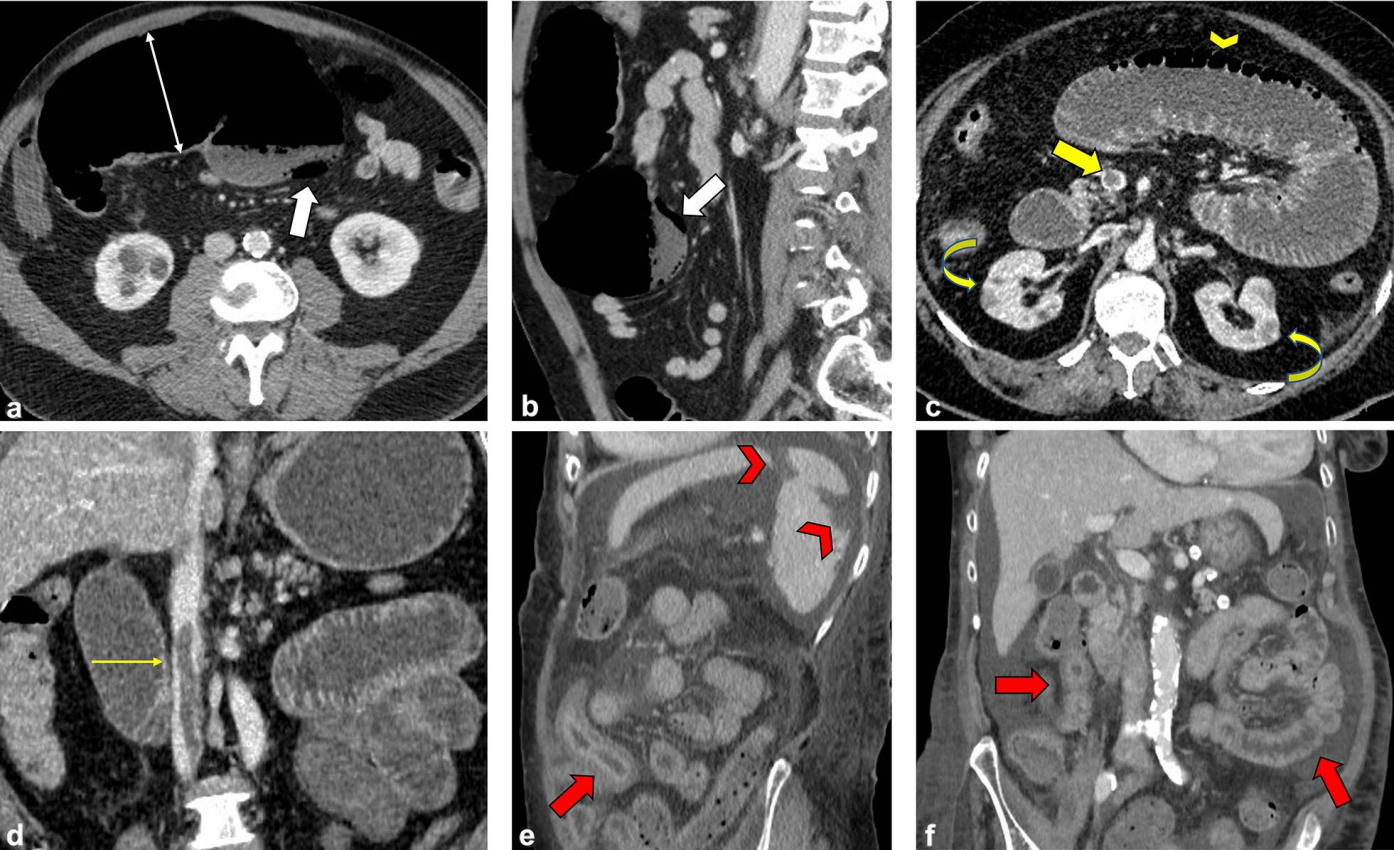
Representative images of abdominal ischemic complications. Computed tomography scans from COVID-19 patients with ischemic complications. A, B Axial and sagittal CT images of a 63-year-old patient show evidence of ischemic colitis involving the transverse colon which looks overdistended (white thin arrow, A), with thin non-enhancing walls and evidence of pneumatosis coli (white arrows, A and B). The main mesenteric vessels were patent (not shown). C, D Axial and coronal CT images of a 62-year-old patient revealed superior mesenteric vein thrombosis (yellow arrow, C) and dilated small bowel loops with pneumatosis intestinalis (yellow arrowhead, C). Concomitant signs of renal infarctions were also noted (yellow curved arrows, C). The same patient also showed inferior cava vein thrombosis (thin yellow arrow, D). E, F Sagittal and coronal CT images of a 79-year-old patient with markedly thickened and layered small bowel walls (red arrows, E and F) associated with multiple peripheral splenic infarcts (red arrowheads, E) with areas of mottled increased attenuation; the main splenic vessels were patent (not shown)
